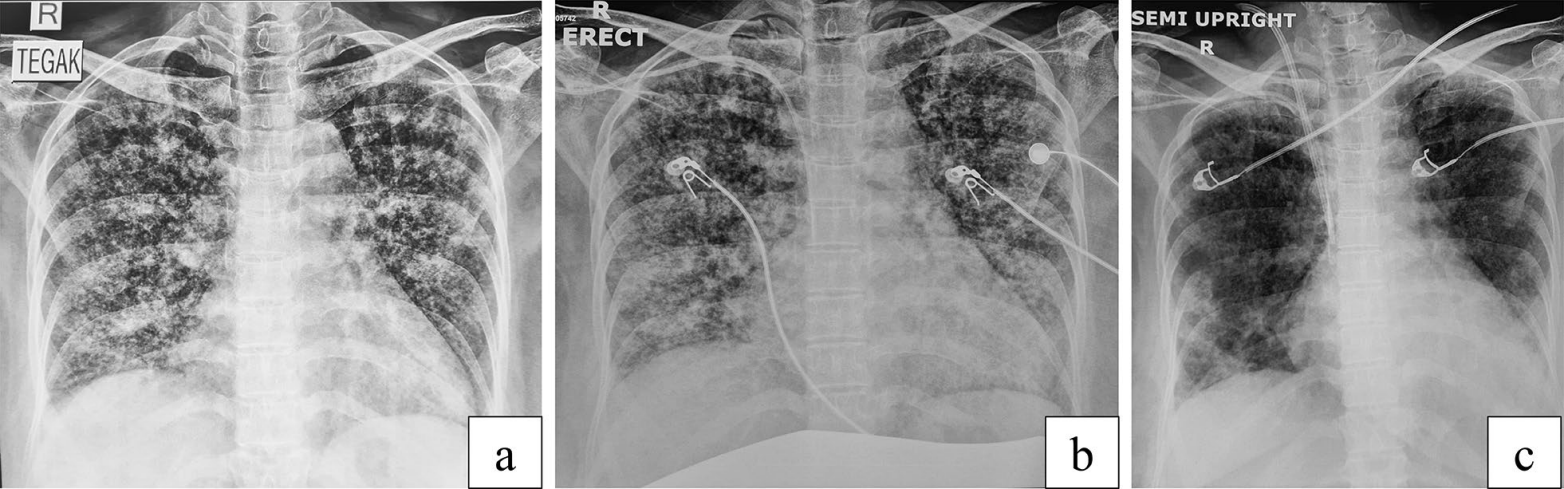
Chest x-ray when admitted (a), before continuous veno-venous hemofiltration (b), and after continuous veno-venous hemofiltration (c)
